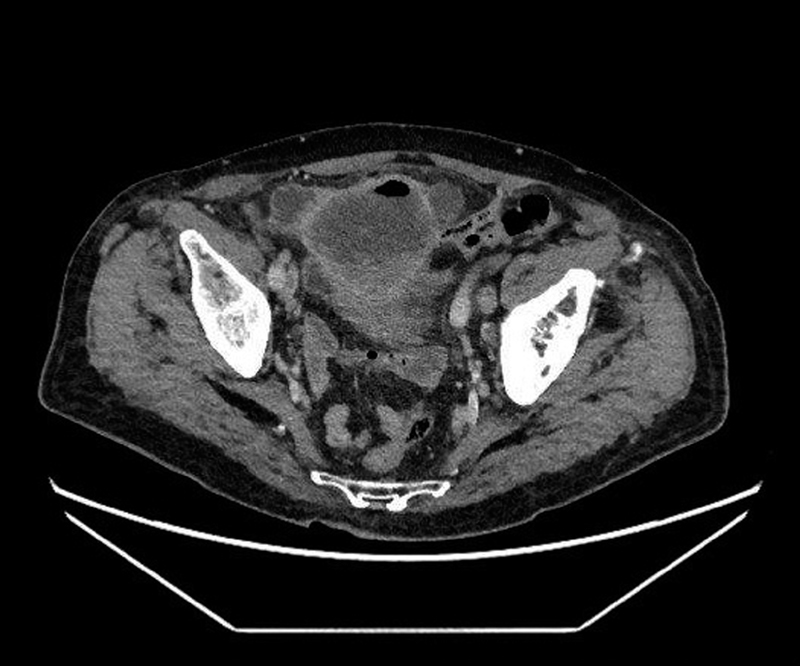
Computed Tomography image of complex mass involving the urinary bladder and small intestine.
